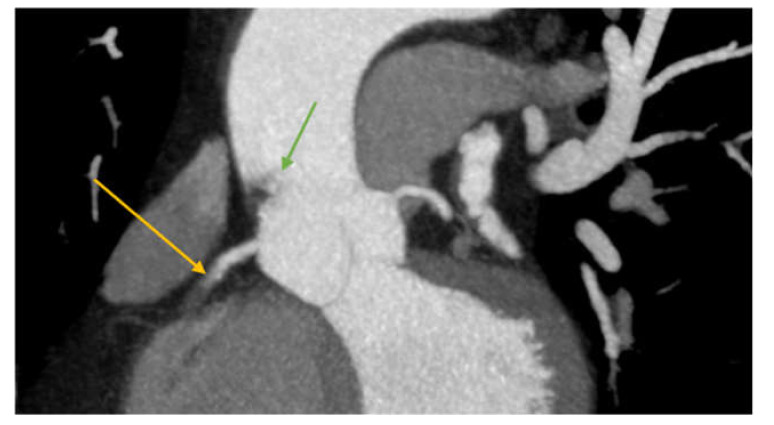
CTA image showing right coronary artery obstruction (yellow arrow) and the endoluminal thrombus (filling defect in the ascending aorta—green arrow).
